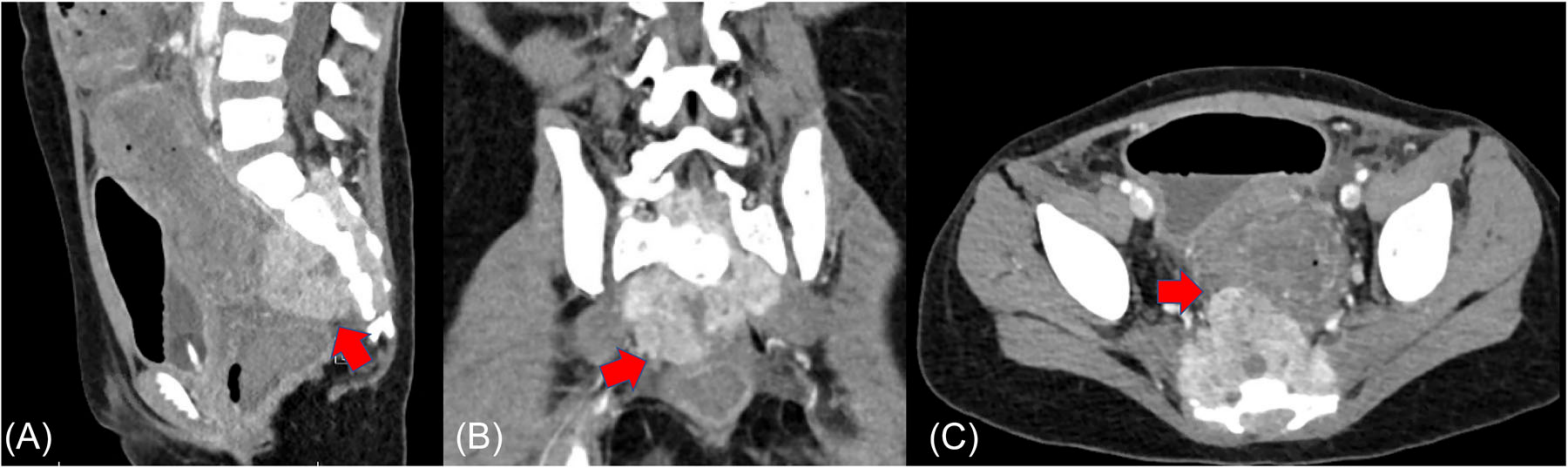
Preprocedural CT demonstrates sacral metastasis (red arrows) with circumferential extraosseous tumor extension into the posterior aspect of the adjacent rectum (yellow arrows). (A) Sagittal. (B) Coronal. (C) Axial. CT, computed tomogra.
